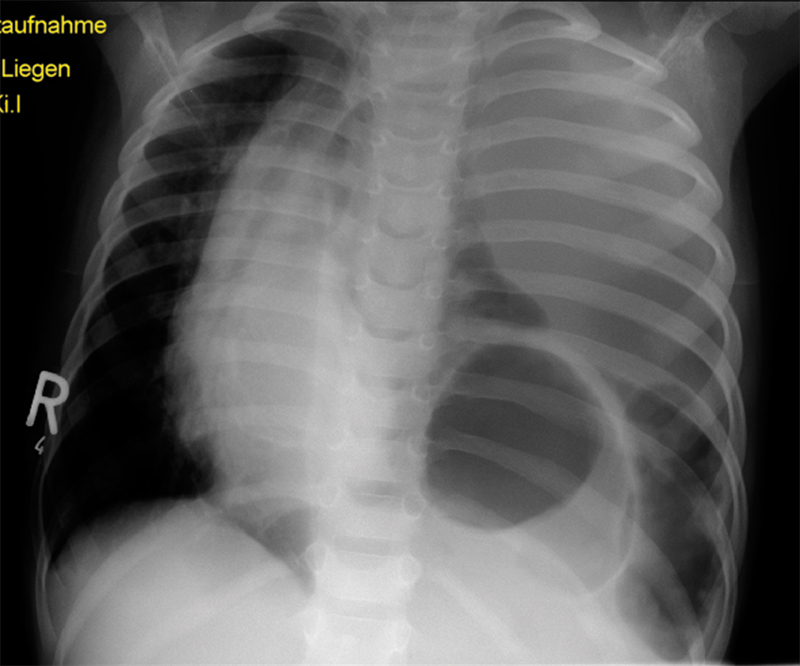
Chest X-ray at presentation (this image is provided by courtesy of Pediatric Clinic, Fachkrankenhaus Hubertusburg, Wermsdorf, Sachsen, Germany).
